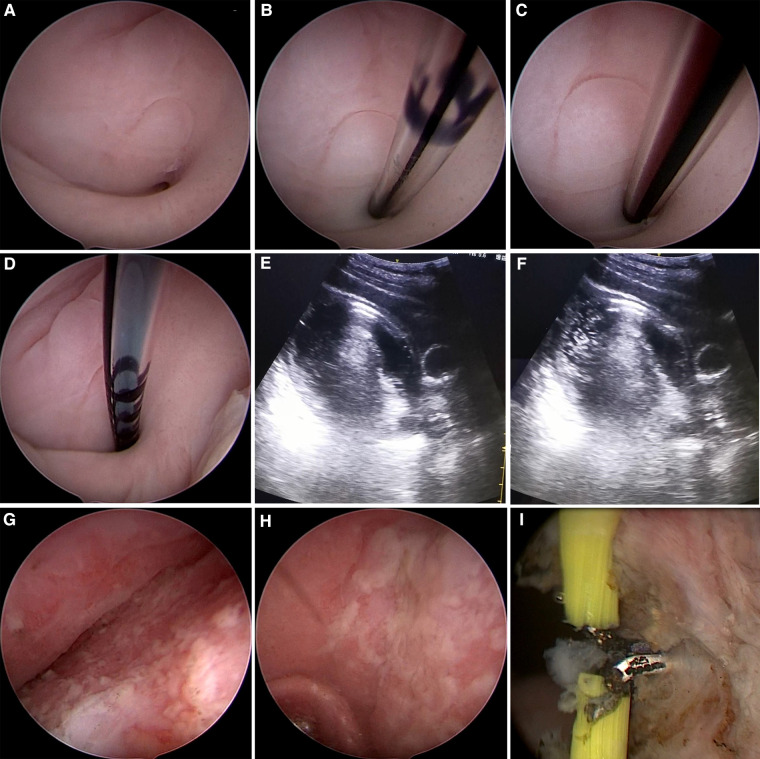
Hysteroscopic and ultrasonic images of the patient during operation. (A) A small hole with a diameter of approximately 1.5 mm was seen at approximately 6 o’clock of the top of the vagina. (B) An epidural catheter was inserted through the hole. (C) Dark red fluid was observed on suction. (D) An injection of 20 ml methylene blue was administered through the epidural catheter. (E,F) A fluid flow shadow in the vaginal mass was observed after injection of methylene blue under ultrasonography. (G,H) A large amount of sloughy pus was observed attached to the vaginal wall and cervix. (I) A needle electrode was used to release the adhesion to the vaginal wall longitudinally.
